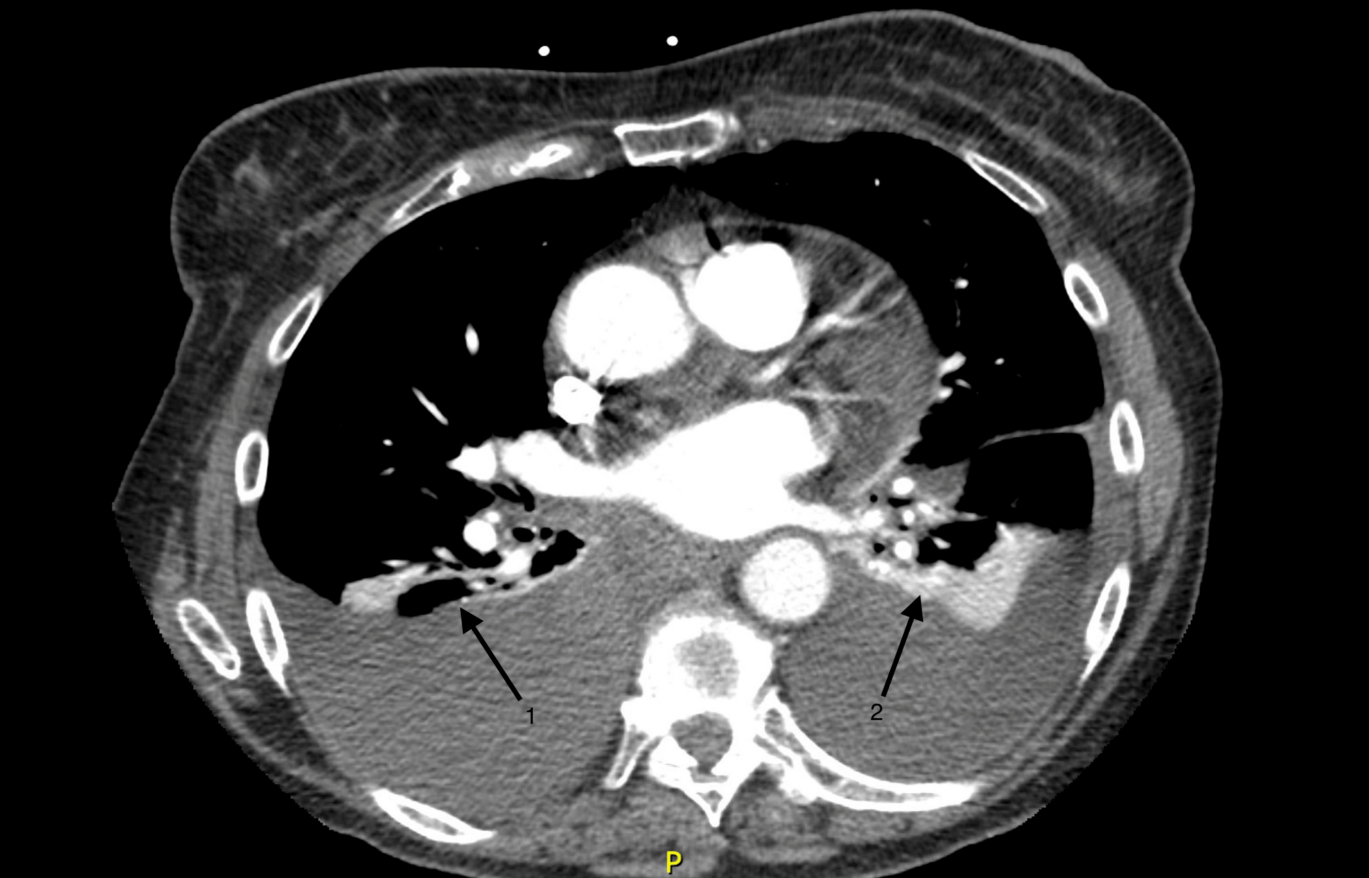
CT chest angiogram Arrows 1 and 2 show bilateral pleural effusions. No pulmonary embolism was visualized CT: computed tomography
